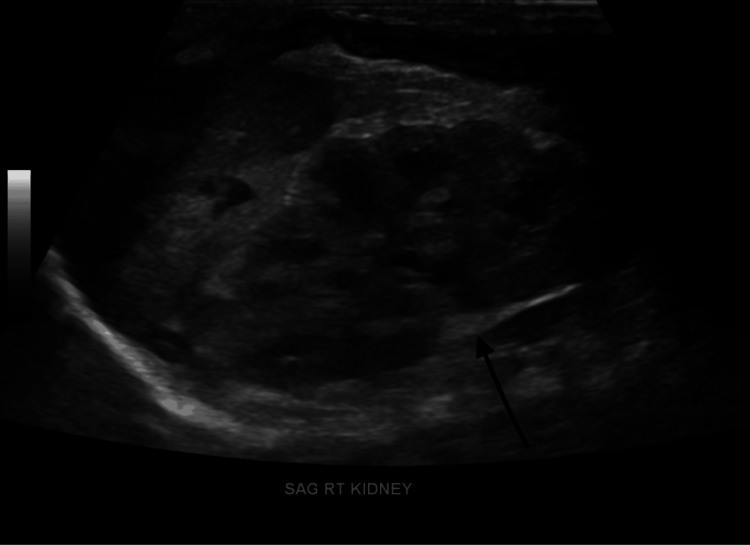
Ultrasound of the Right Kidney Ultrasound showing mild right-sided hydronephrosis.
